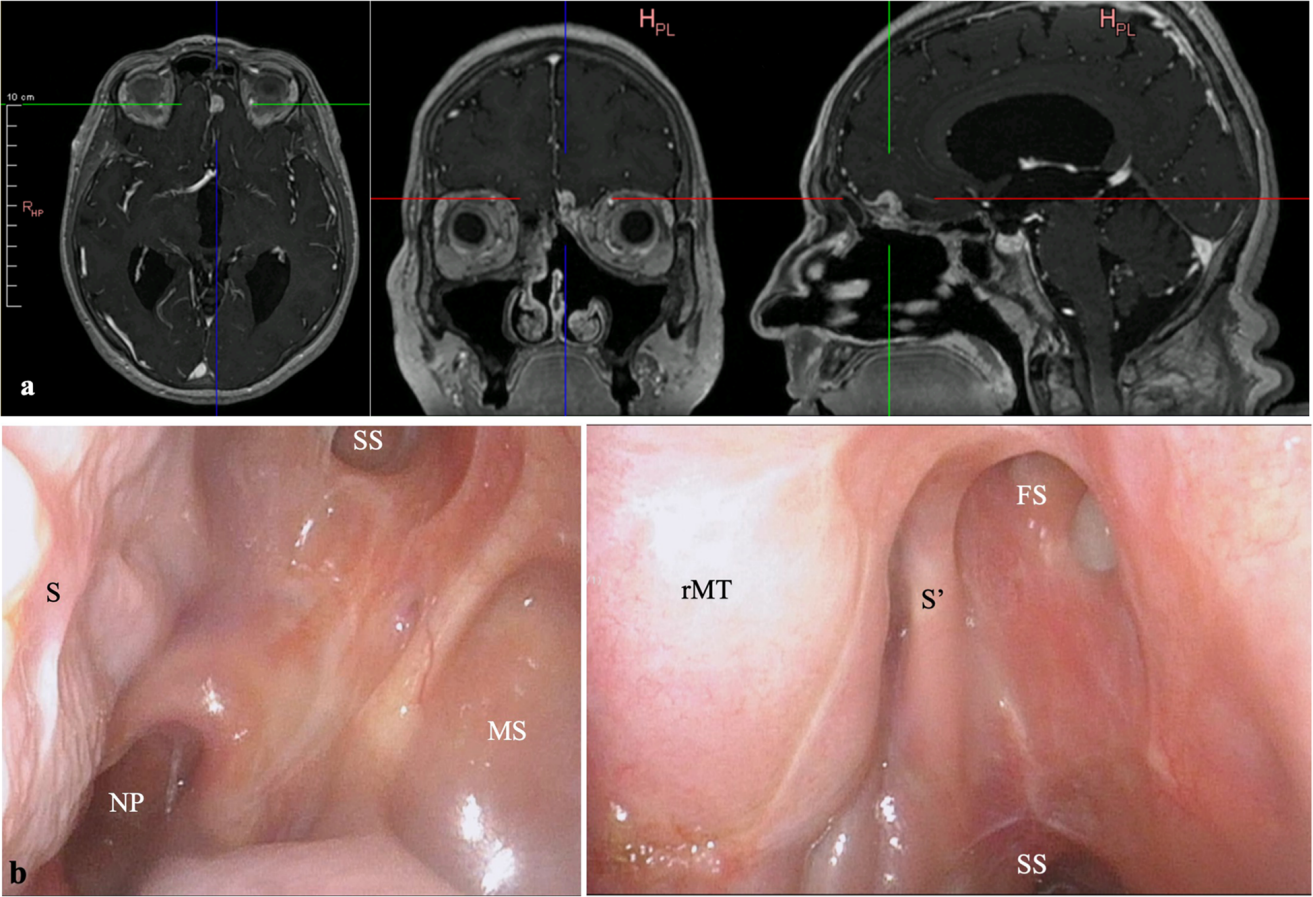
Follow-up 16 months postoperatively: MRI revealed an enhancing mass at the left anterior frontal skull base and the ethmoid roof, displaying a heterogeneous signal on T1- and T2-weighted sequence suspected of residual tumor, which did not correlate with the clinical and laboratory outcomes of the patient (a). Endoscopic view of the operation field with no sign of recurrence. Nasal septum (S), nasopharynx (NP), sphenoid sinus (SS), maxillary sinus (MS), right middle turbinate (rMT), partially resected nasal septum (S’), frontal sinus ostium with a small-sized polyp (FS) (b, c)
